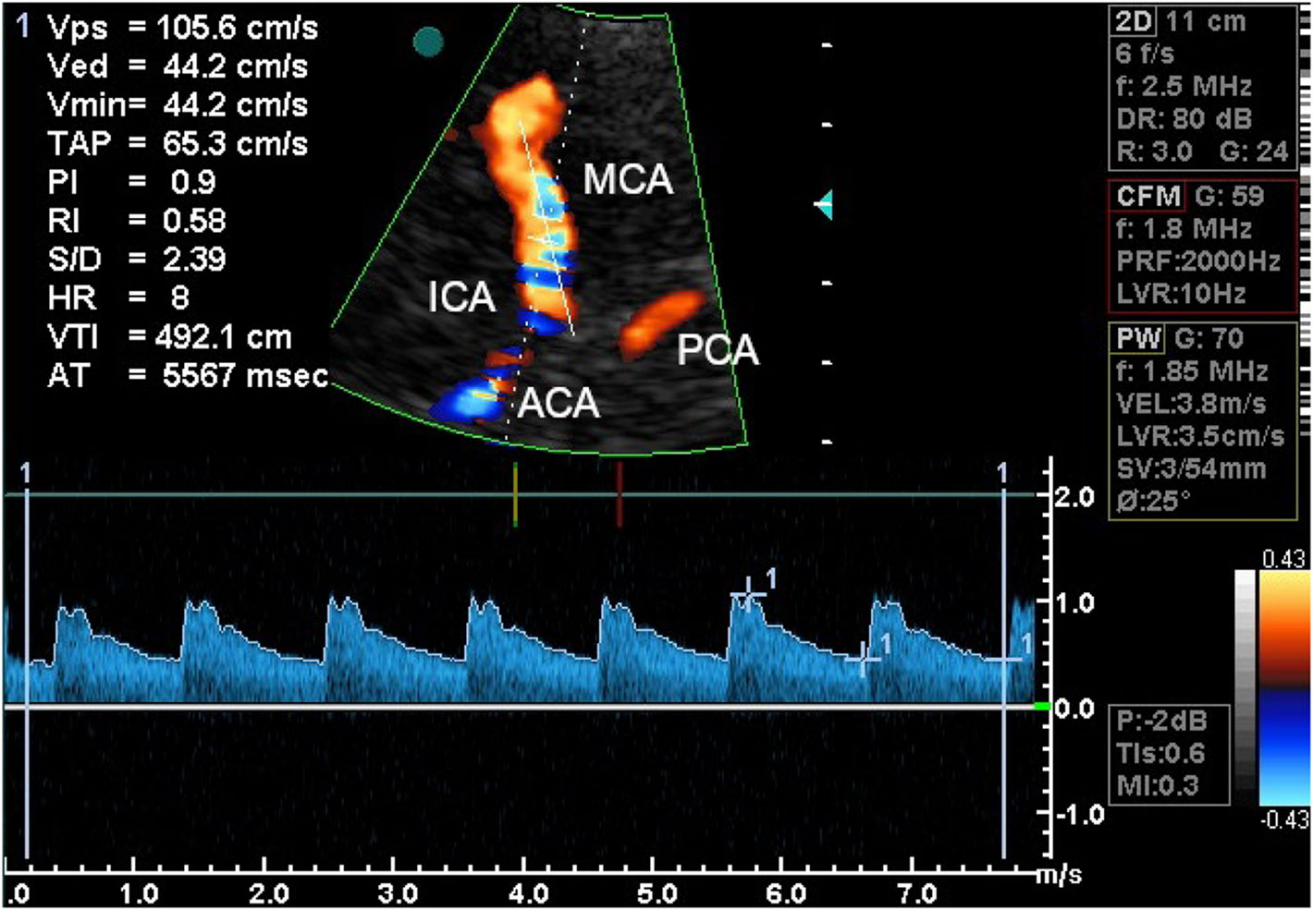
View of a colour-coded image of the middle cerebral artery (MCA) with a corresponding Doppler spectral analysis performed using a transtemporal insonation. The sample volume was placed at a depth of 54 mm in the distal portion of the MCA. ICA – the internal carotid artery; PCA – the posterior cerebral artery; A1 ACA – A1 segment of the anterior cerebral artery
